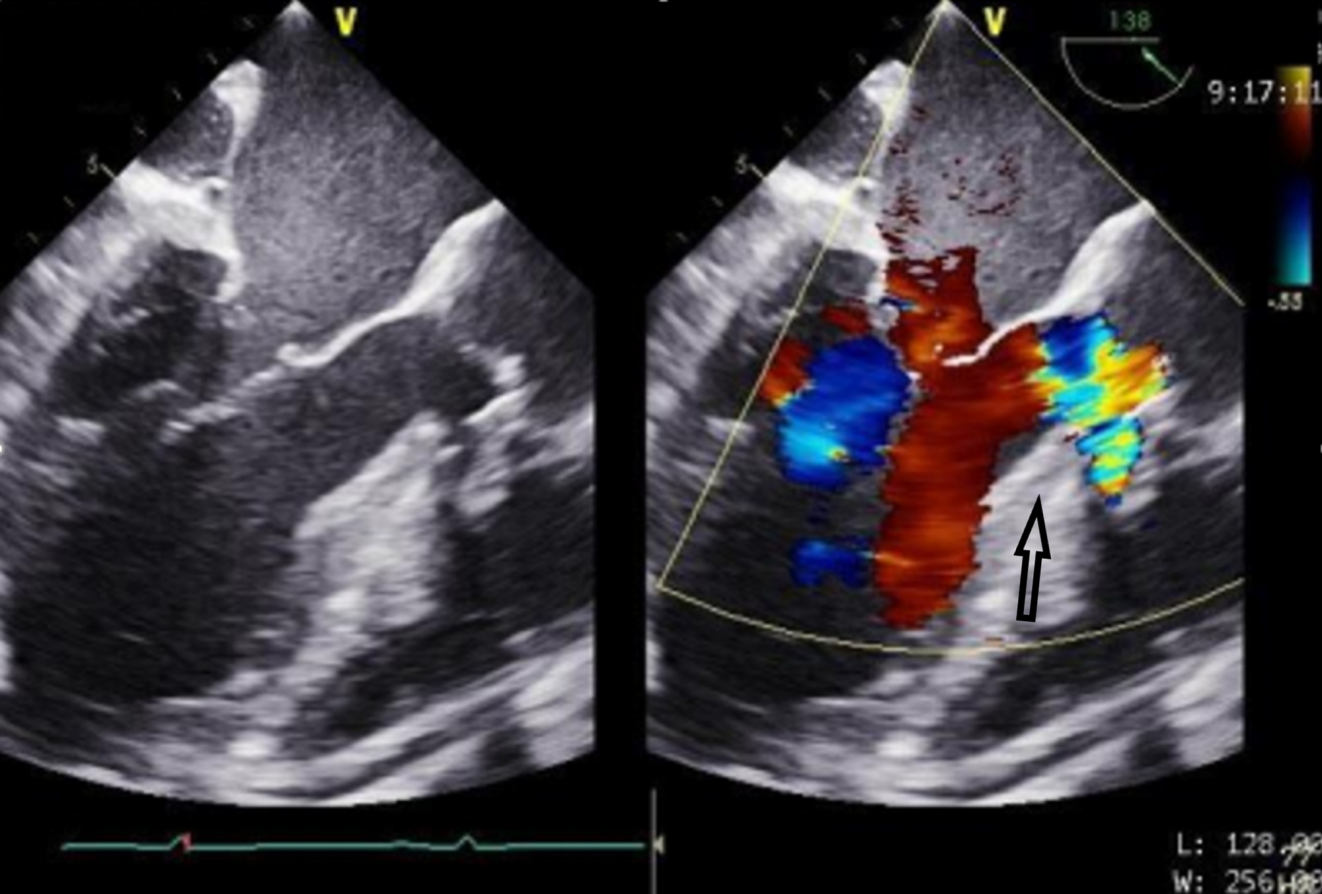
Midesophageal long axis view depicting the left atrium (top) and LV (bottom). Color flow doppler is used on the right figure to demonstrate the severity of AI (arrow). LV, left ventricle; AI, aortic insufficiency
